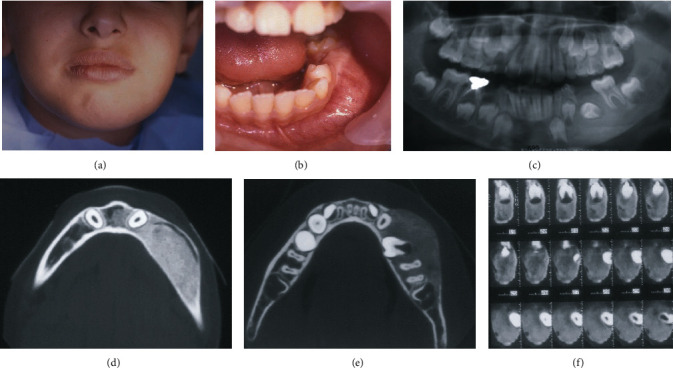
(a) Facial asymmetry on the left side. (b) Swelling in the buccal side of the mandible. (c) Panoramic X-ray showing the radiopaque image englobing the dental germ of the permanent second premolar. (d) The course of the mandibular canal displaced buccally is shown on the axial image of the CBCT. (e) Axial image of the CBCT showing the expansion of the body of the mandible. (f) Para-axial images of the CBCT revealed the ossification of the lesion and the positions of the retained dental germ and the mandibular canal.
